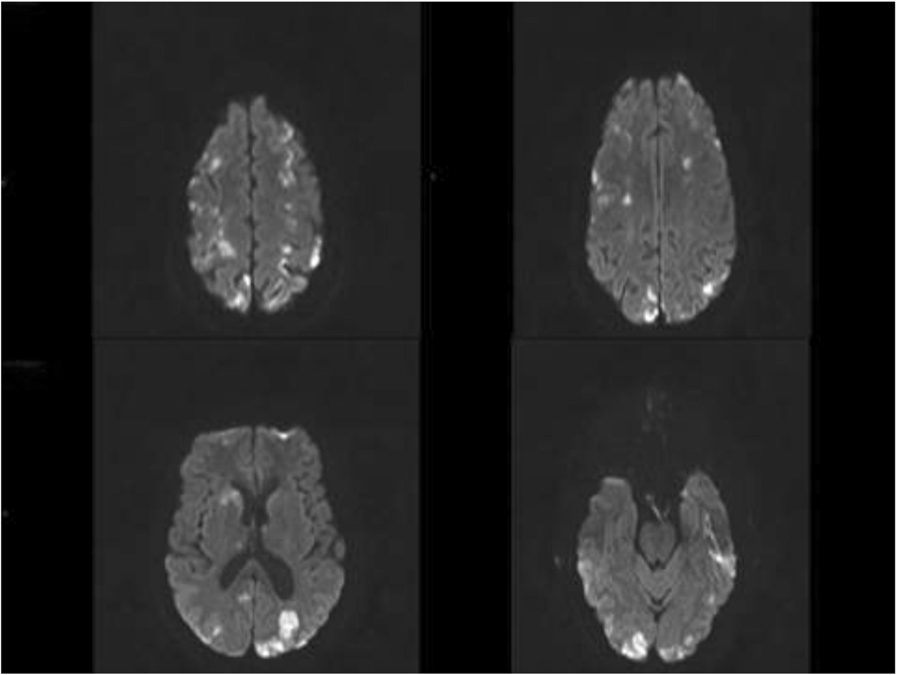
Magnetic resonance imaging of brain (diffusion weighted imaging sequences) in a patient with severe left ventricular systolic dysfunction and acute cognitive change. The initial images were felt to represent a multi-infarct state, presumed cardioembolic and 'watershed' (hypoperfusion) infarction. Subsequent investigations revealed that the patient had 'shared' cardiac and cerebral pathology caused by a systemic and cerebral vasculitic process.
